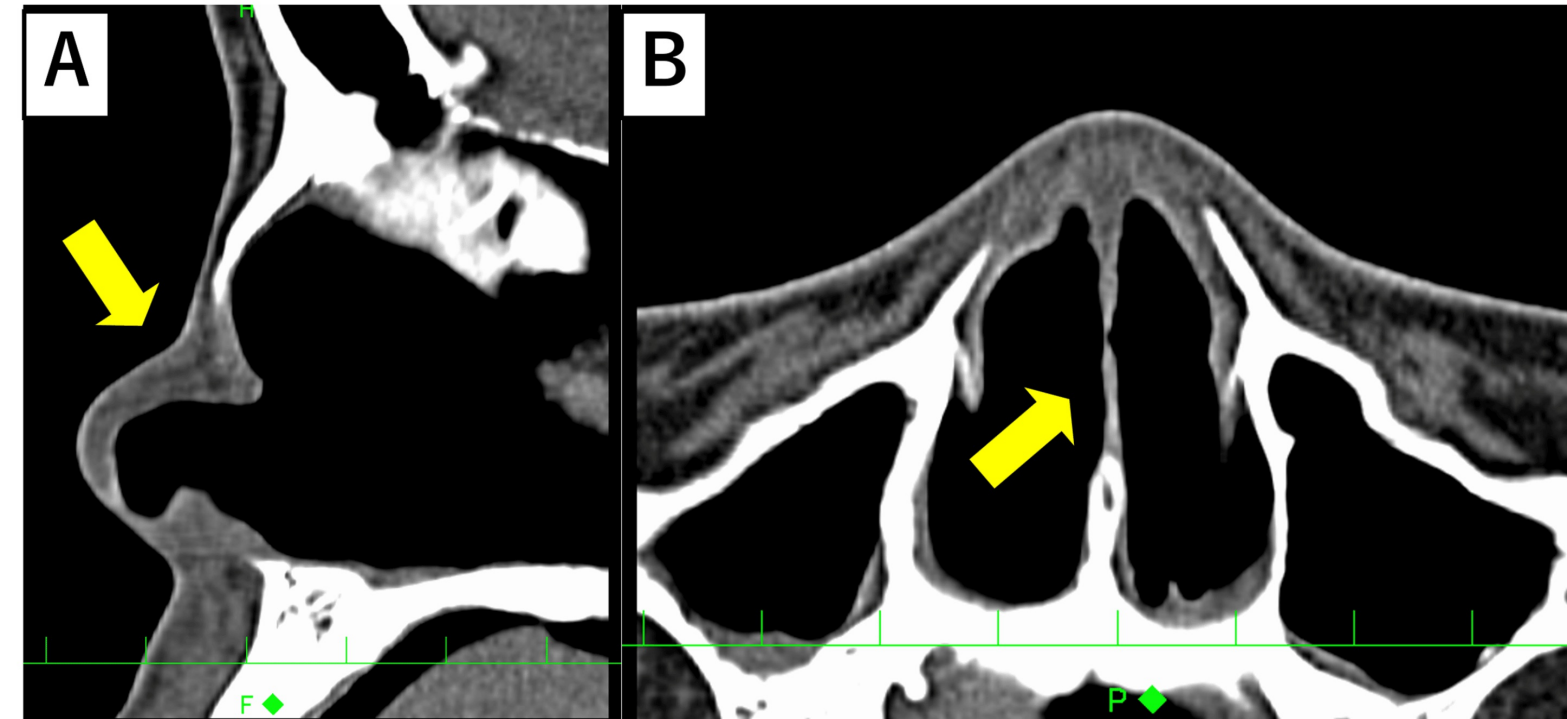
CT findings in Case 2, showing skin, mucosa, and cartilage thinning (yellow arrow) (A) Sagittal view. (B) Axial view
